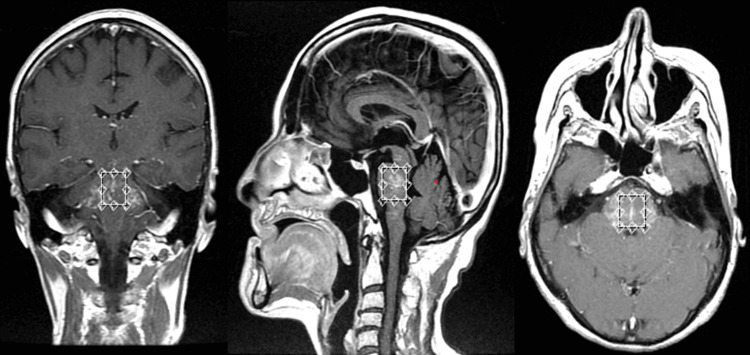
MRI of the patient MRI showing hyperintense images at the level of the midbrain, pons, and both cerebellar peduncles and medulla, revealing isointense behavior in T1 with avid post-contrast enhancement MRI: magnetic resonance imaging
